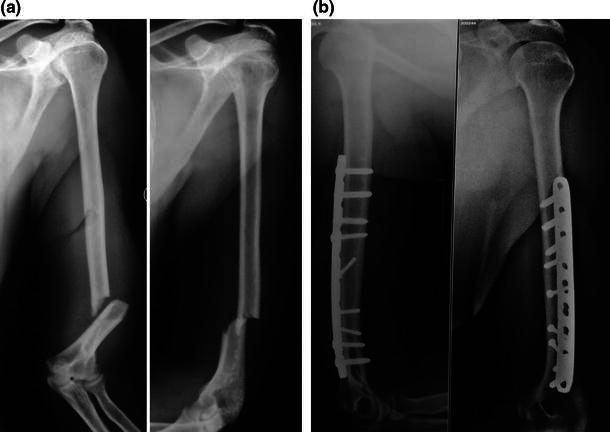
a Anteroposterior and lateral views showing a transverse fracture of the distal third of the humerus. b Post-operative anteroposterior and lateral radiographs showing sound union at 5 months following fixation with LCP
